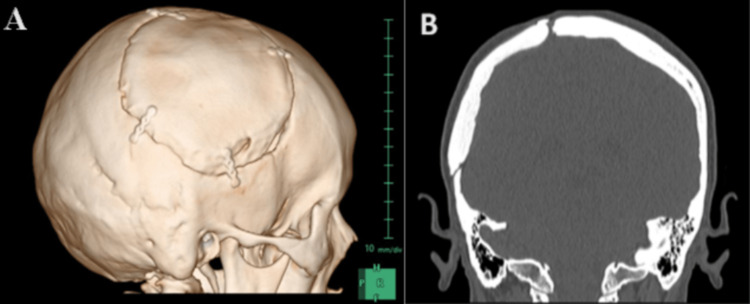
Follow-up head CT scan taken four years after tumor excision and cranioplasty (A) 3D reconstructed CT head. (B) Coronal view. Both images demonstrate the right parietal cranioplasty with no recurrence of either of the bone tumors.
